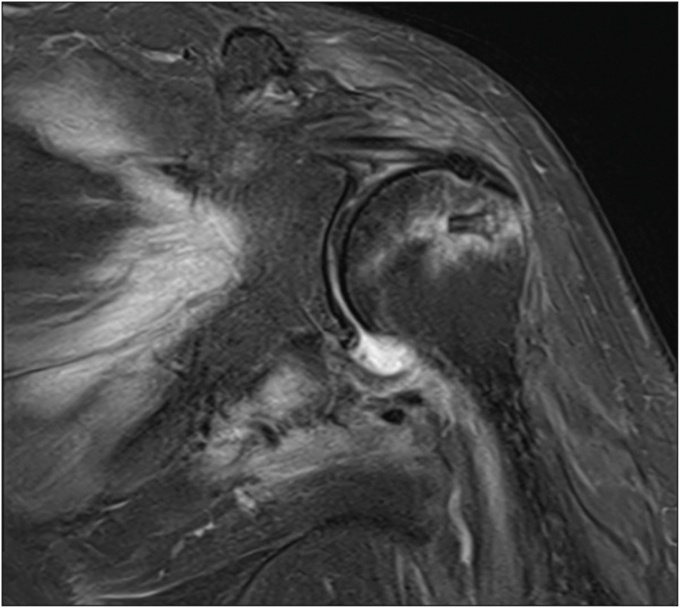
Post-reduction MRI of the left shoulder showing no evidence of rotator cuff re-tear, confirming the integrity of the initial repair. *MRI*, magnetic resonance imaging.
